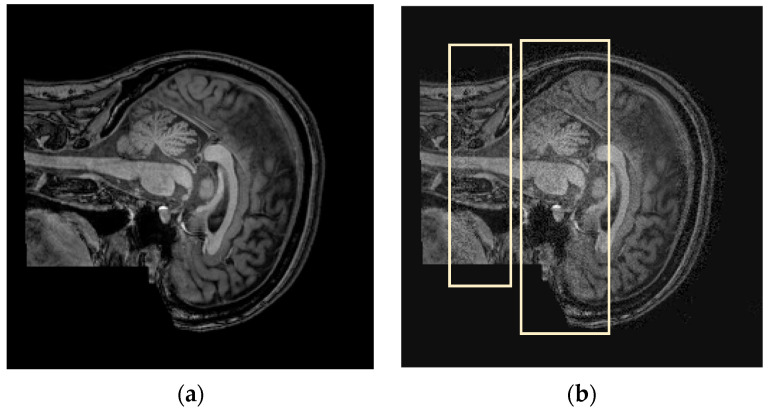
Zipper artifact: image appearance. (a) Artifact-free brain MRI. (b) Image with the zipper artifact; rectangles show the vertical noise patterns.
